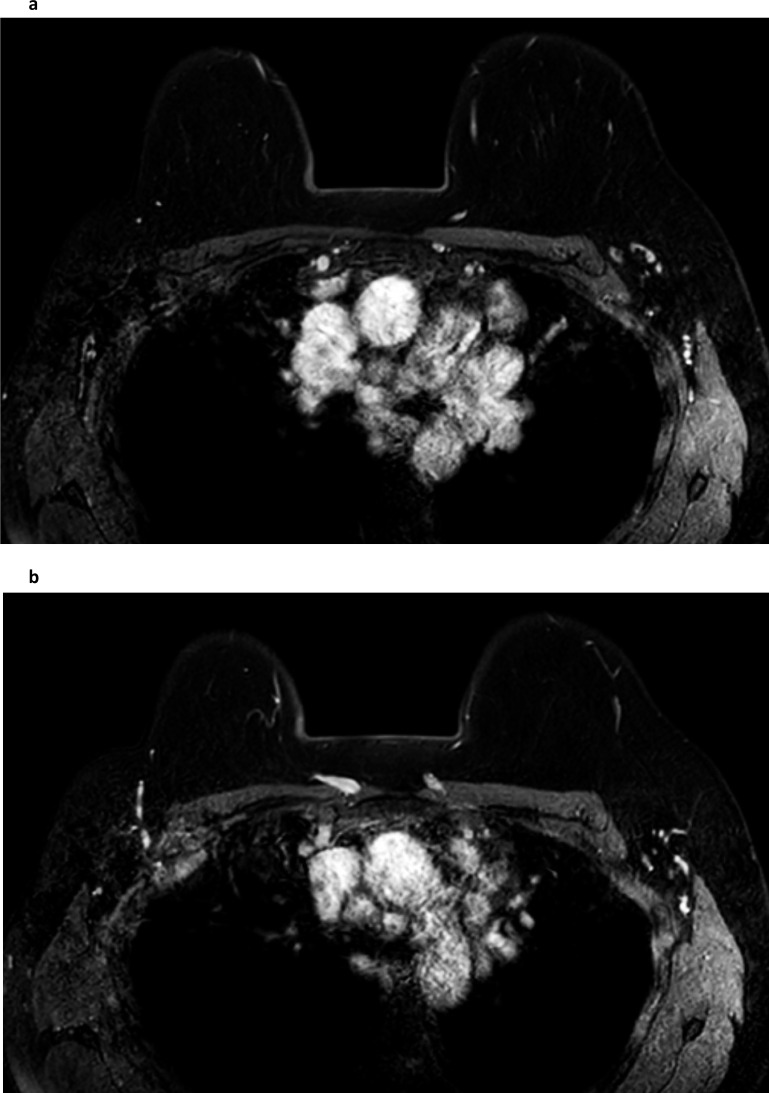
Incidental mediastinal lymphadenopathy. 4 years after a high-risk screening client had right breast conserving surgery and radiotherapy, her breast MRI demonstrated mediastinal lymphadenopathy, shown here on two slices from the post contrast dynamic series (a and b). Following investigation, a new diagnosis of sarcoid was made. These images are of the same patient as Figure 3.
